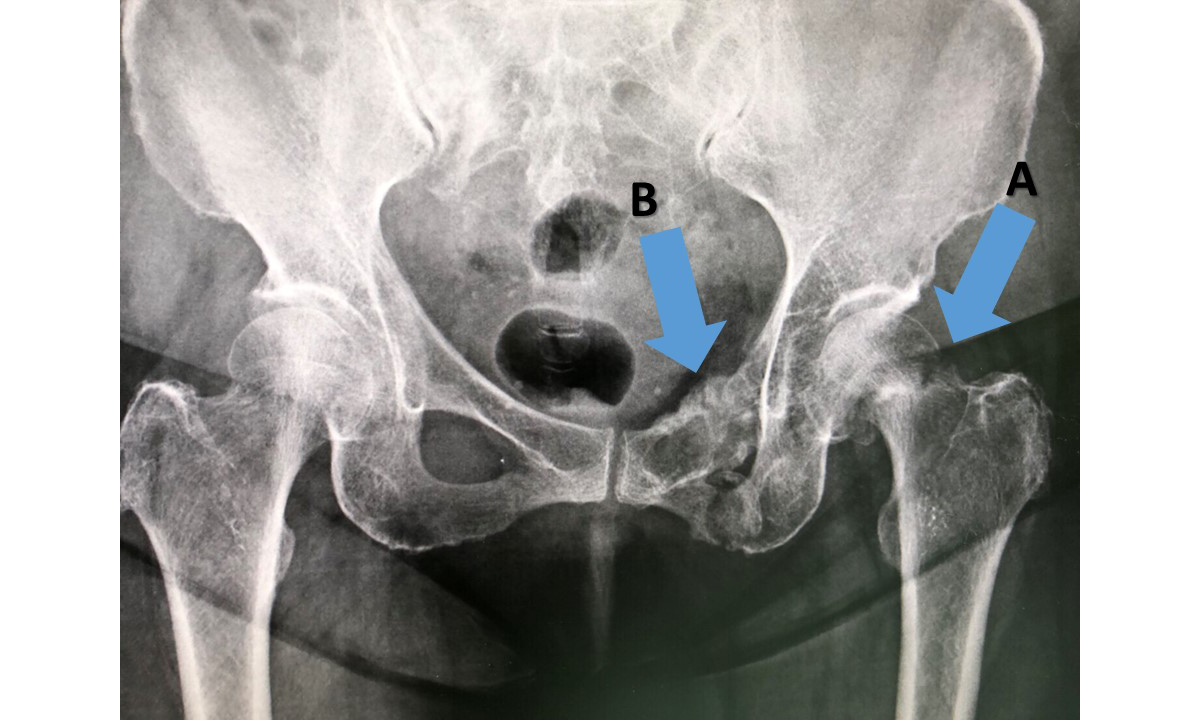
Plain radiograph image, which is used in task 5, showing fractured neck of left hip (femur) (A) and pelvis (pubic rami) (B).
